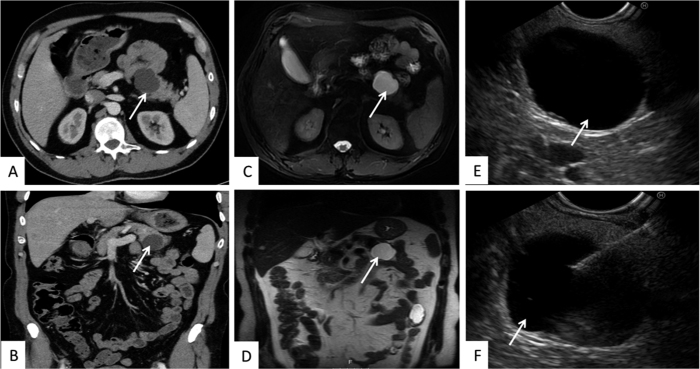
Pancreatic body cysts as detected by CT (A,B), MRI (C,D), EUS (E) and EUS-FNA (F). Arrows indicate the location of the pancreatic cyst.
